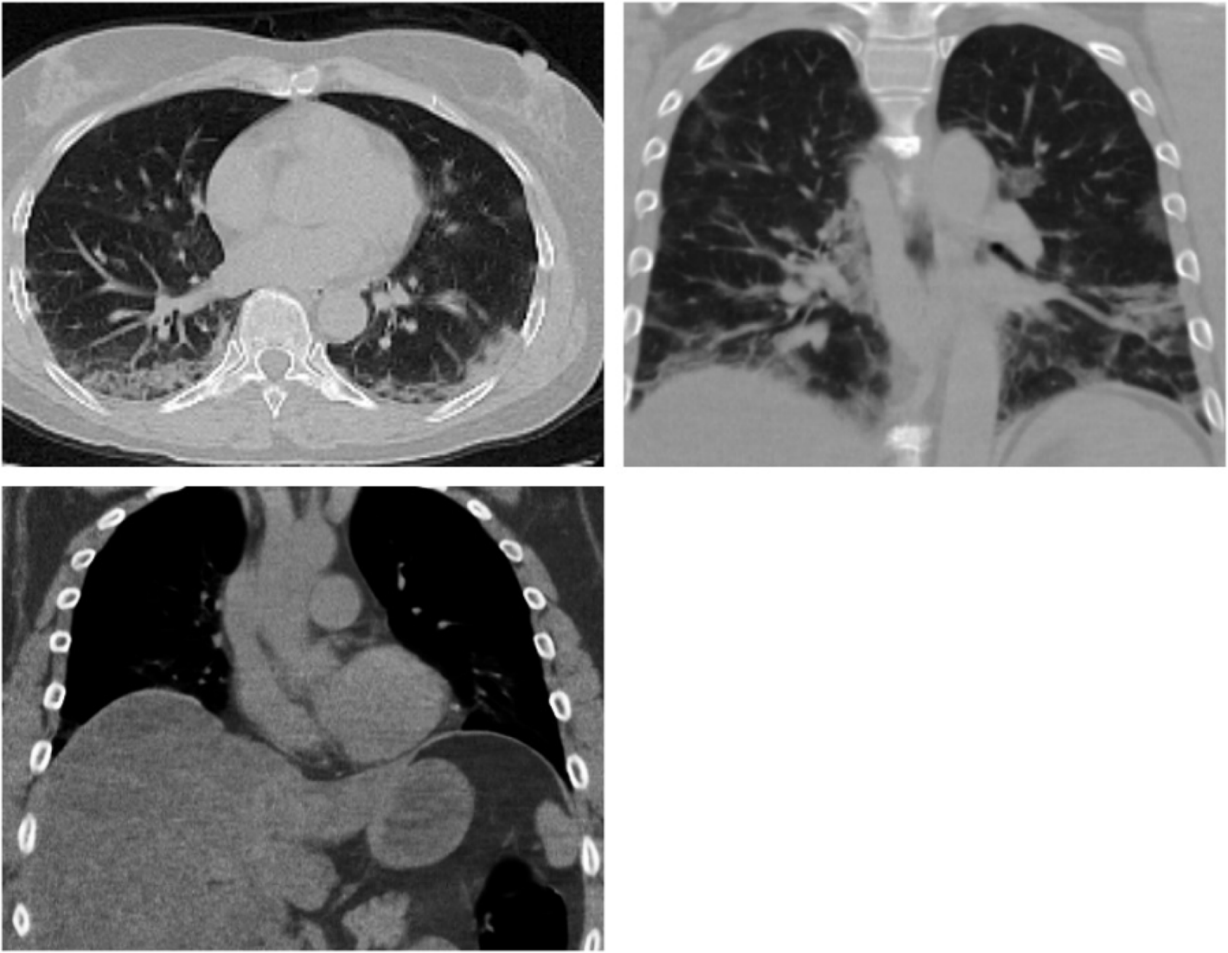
Male patient, 64 years. CT chest shows bilateral lower lobar subpleural patchy ground-glass opacities with underlying interlobular septal thickening and atelectatic bands, reported as CORADs 5. Upper abdominal cuts show fatty hepatomegaly
